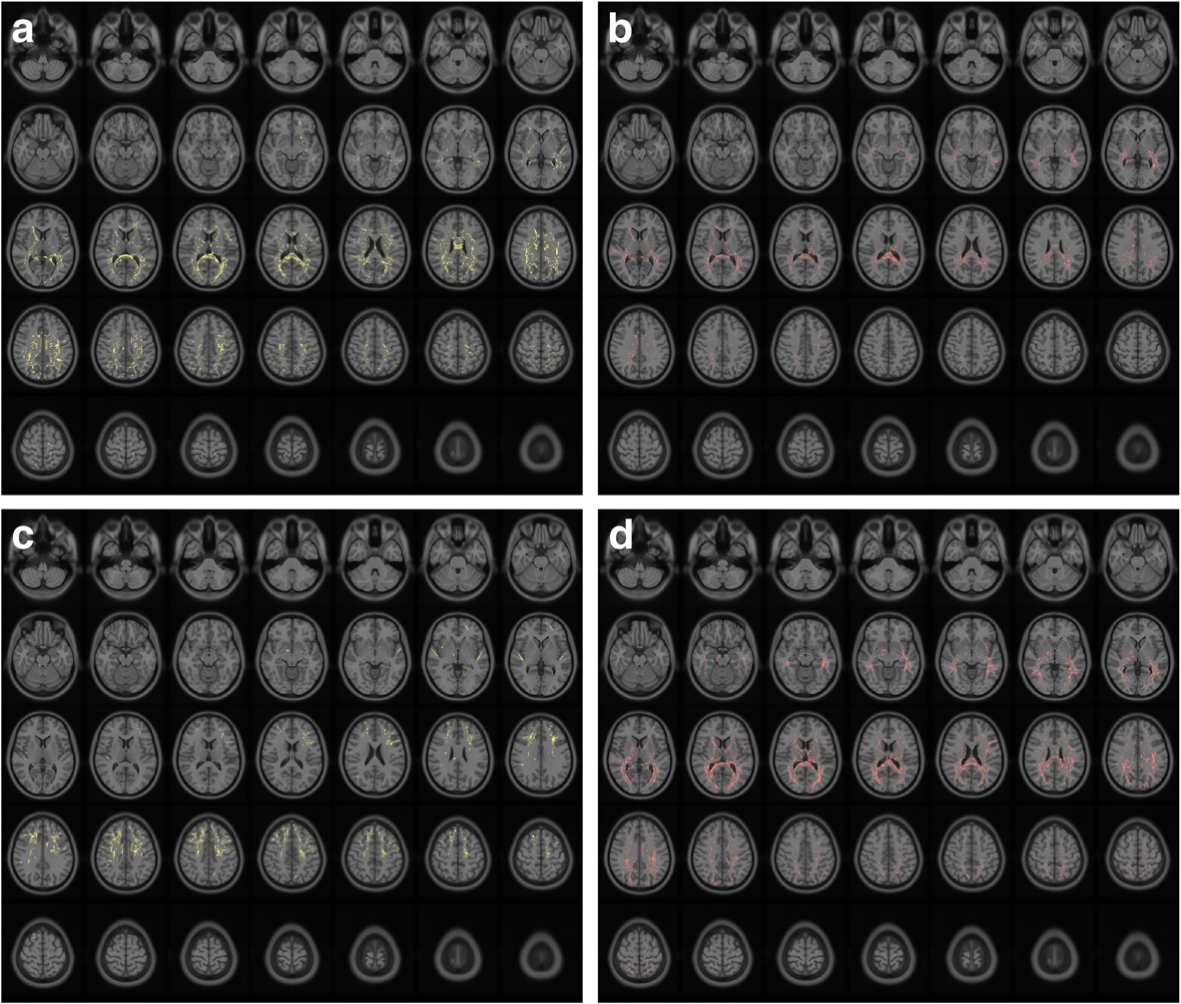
Results of the diffusivities measures (fractional anisotropy and radial diffusivity) analysis comparing 23 patients with TN wNVC and 22 patients with TN wNVC and 45 healthy controls. Results of the statistical analysis are overlaid on an axial T1 anatomical magnetic resonance imaging template
